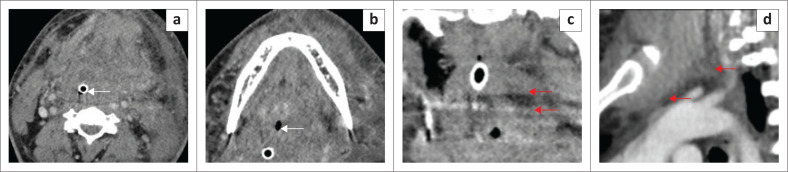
A case of Ludwig’s Angina in an adult patient who presented with acute stridor, difficulty swallowing and marked swelling in the left upper neck. The patient was intubated. CT neck with contrast was partly degraded by artefacts from dental amalgam. There was extensive soft tissue thickening of the floor of the mouth (FOM) (a, b) with the loss of the normal anatomical boundaries at the FOM and other neck spaces, marked narrowing of airspaces (b, white arrow) and evidence of intubation (a, white arrow). There was an irregular left parapharyngeal abscess (c, red arrows), which was drained. The sagittal image (d) shows the extension of cellulitis into the anterior mediastinum (red arrows).
